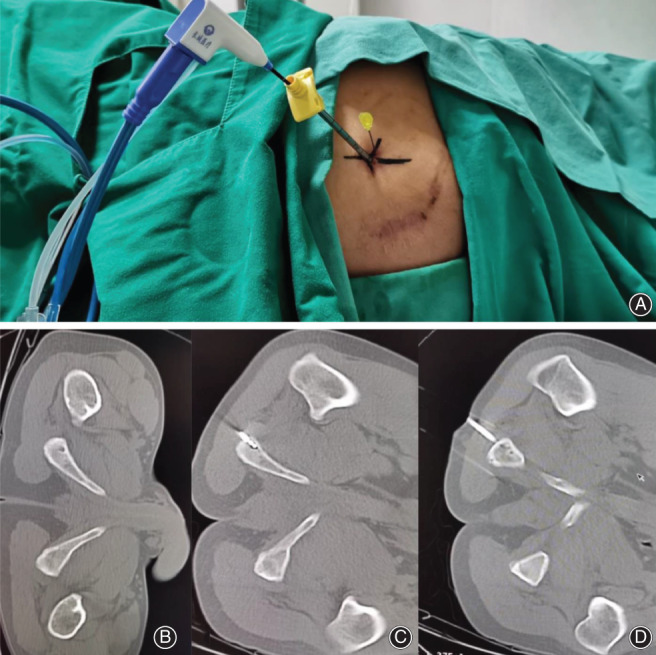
(A) A 37‐year‐old man with liver cancer who received CT fluoroscopy‐guided percutaneous microwave ablation for the treatment of the ischial tuberosity ilium metastasis. (B, C, D) The microwave probe guided by CT and the thermocouple needle is situated in the surrounding normal tissue.
